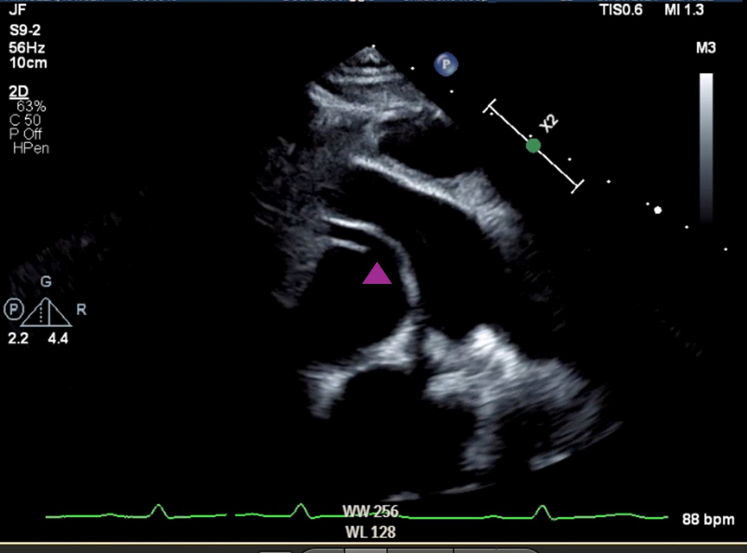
Follow-Up Transthoracic Echocardiogram Transthoracic echocardiogram 2 months after repair, parasternal short-axis view, showing unroofed right coronary artery (purple arrowhead).
